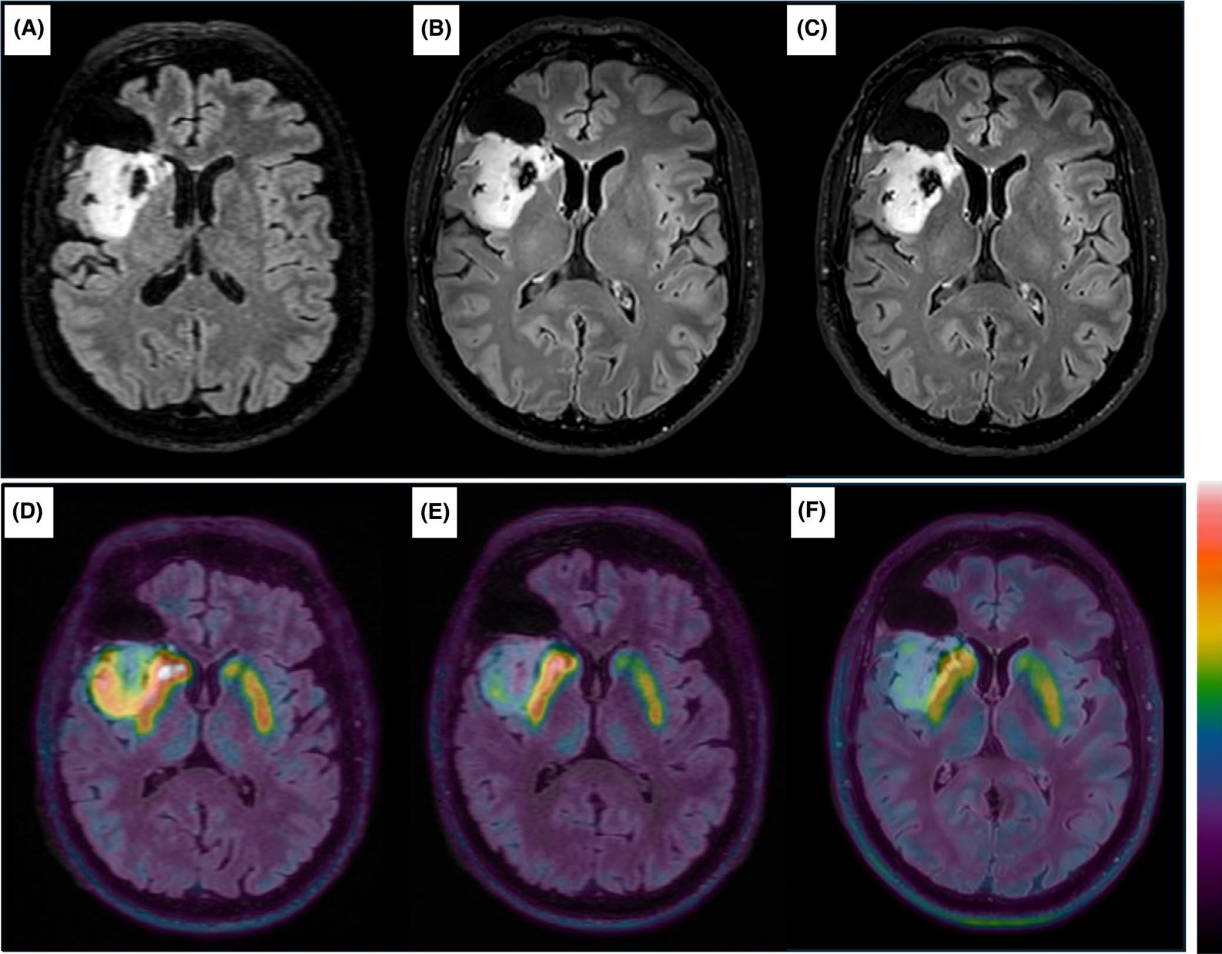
Brain magnetic resonance imaging (MRI) T2/fluid‐attenuated inversion recovery three‐dimensional axial sequences and [^18^F]fluorodopa (F‐DOPA) positron emission tomography (PET) at baseline and after three cycles of vorasidenib. (A) MRI at baseline. (B) MRI after three cycles. (C) MRI after six cycles. (D) [^18^F]‐DOPA PET at baseline. (E) [^18^F]‐DOPA PET after three cycles. (F) [^18^F]‐DOPA PET after six cycles. In PET images, for all timepoints, the maximum uptake of the scale, as presented in the color bar (white), was placed in the region of maximum uptake.
